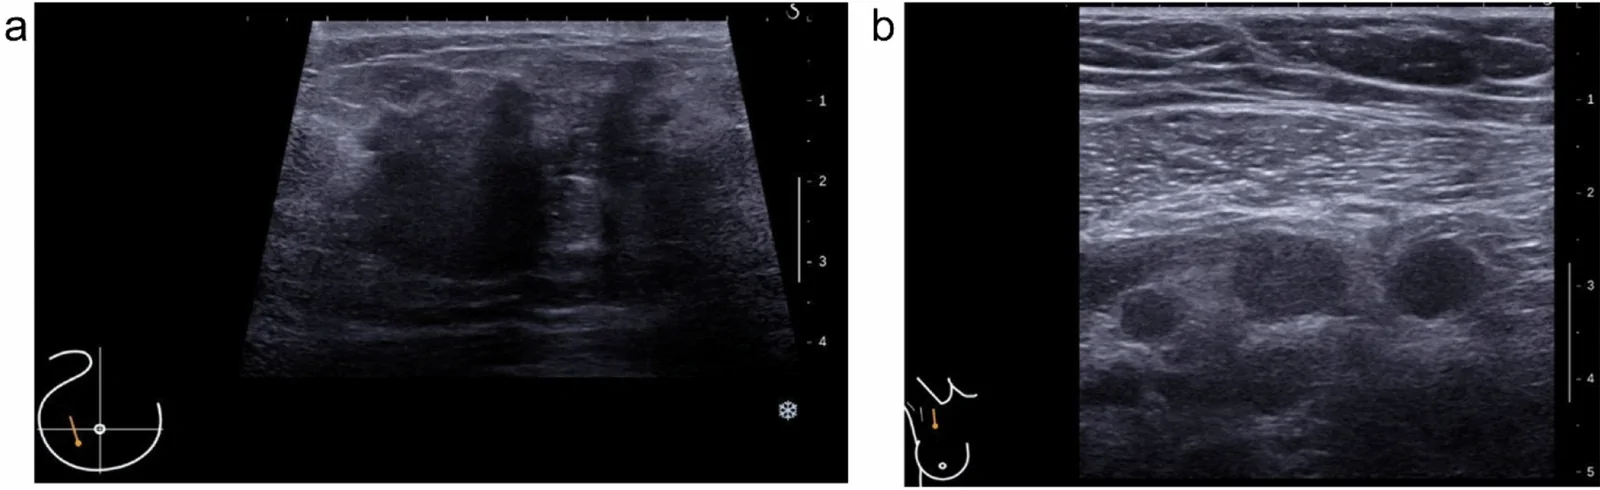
Ultrasonographic findings at presentation. (a) Breast ultrasonography showing a large irregular hypoechoic mass in the right breast; (b) Axillary ultrasonography demonstrating enlarged right axillary lymph nodes suspicious for metastasis
